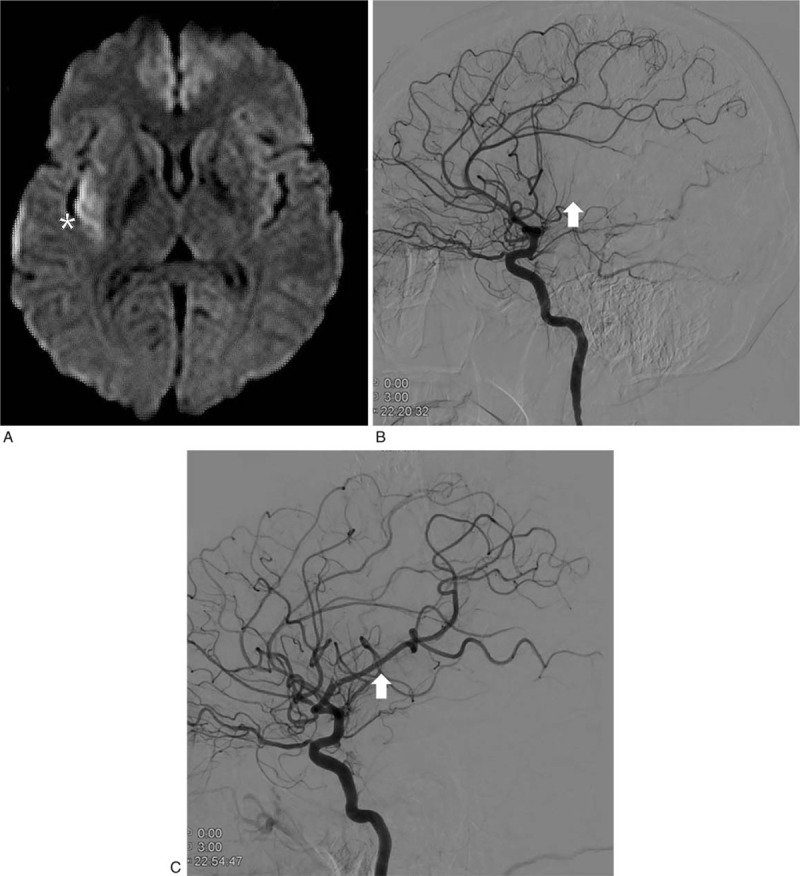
A case of nontraumatic cerebral fat embolism in a young woman (case 1) that presented with right middle cerebral artery occlusion after autologous fat grafting. (A) Diffusion-weighted magnetic resonance imaging reveals localized delay and decrease of perfusion in the right temporoparietal area (asterisk). (B) Trans-femoral cerebral angiography reveals occlusion post-bifurcation of the M1/M2 junction of the right middle cerebral artery (white arrow). (C) Post-interventional angiography highlights successful recanalization (white arrow).
